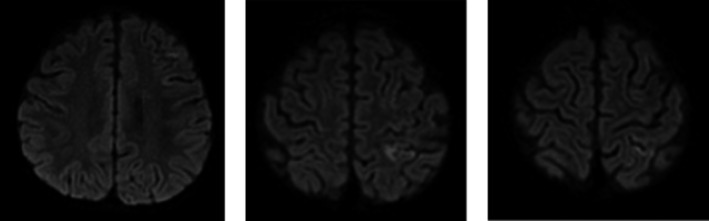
Magnetic resonance imaging of interval near complete resolution of previously seen abnormal restricted diffusion/infarct within the left middle cerebral artery and watershed distribution (day 12 of hospitalization)
